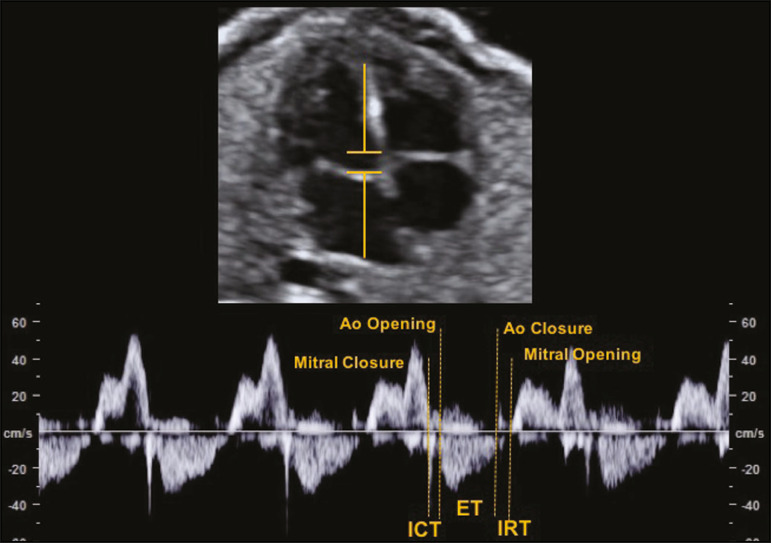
MPI measured by aperture clicks in the Doppler velocity spectrum.
